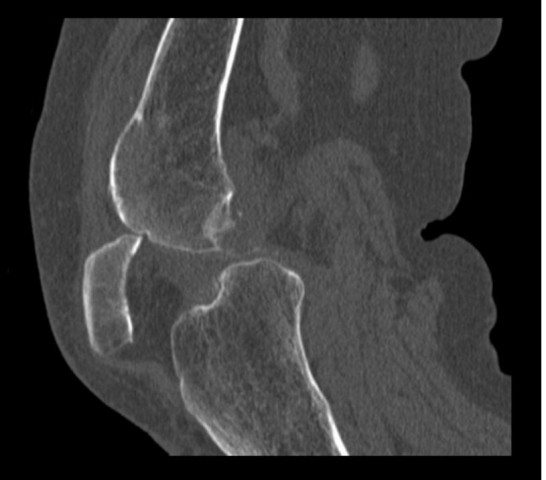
Computed tomography sagittal image showing the superior pole of the patella impacted in the lateral condyle.
